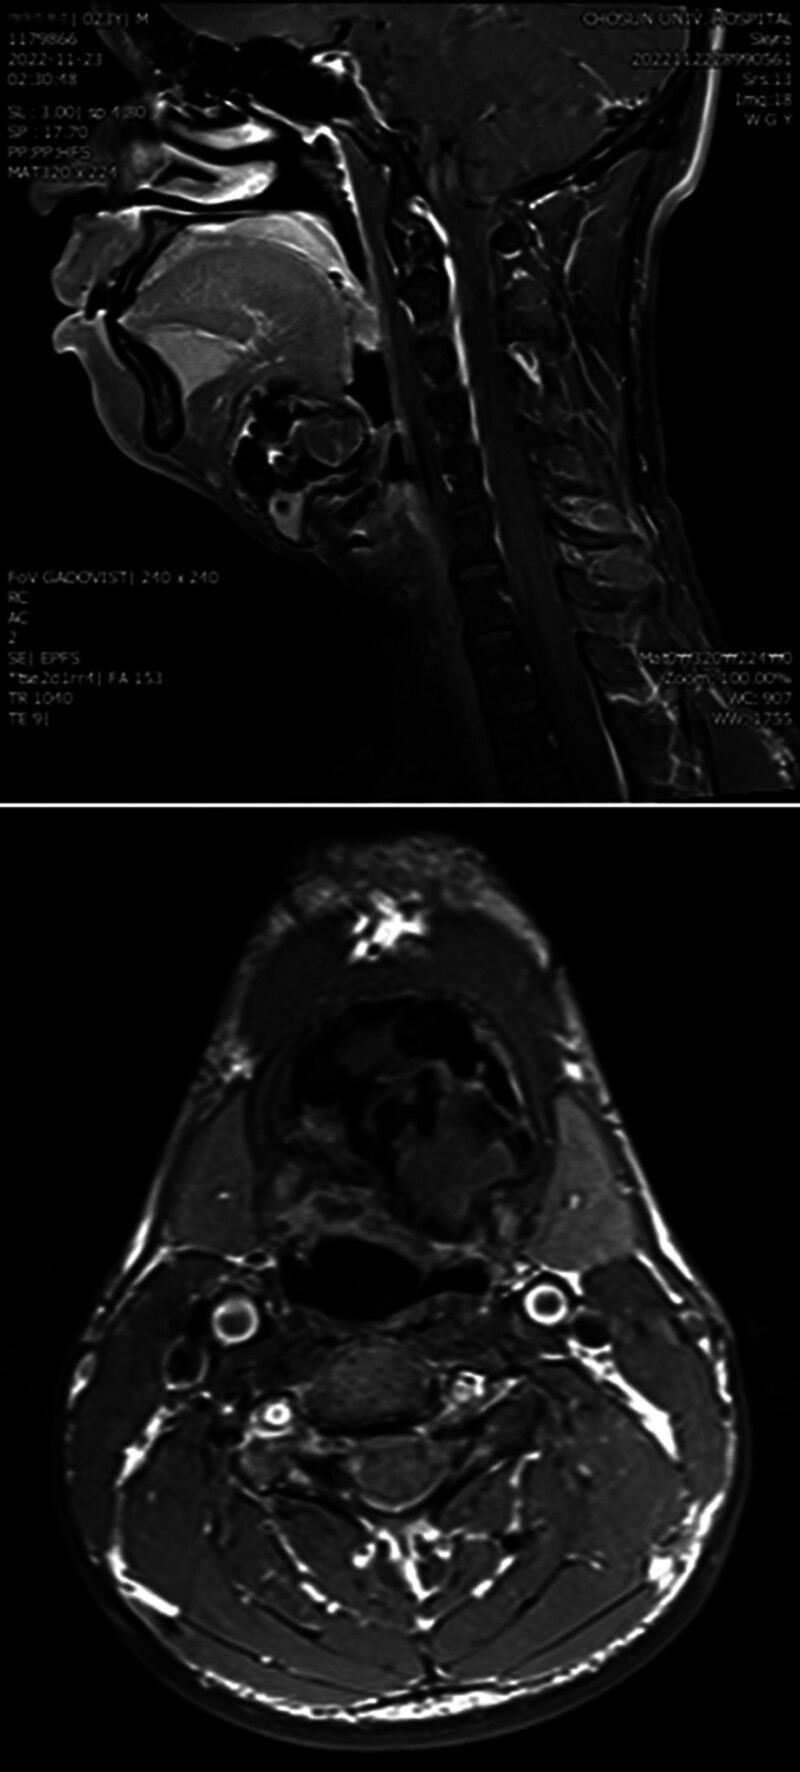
MRI revealing a 5.3 × 4.3 cm mass located in the upper anterior neck. The lesion exhibited mixed signal intensity, consisting of solid components and cystic areas, as depicted in the sagittal view (upper) and axial view (lower) images. MRI = magnetic resonance image.
